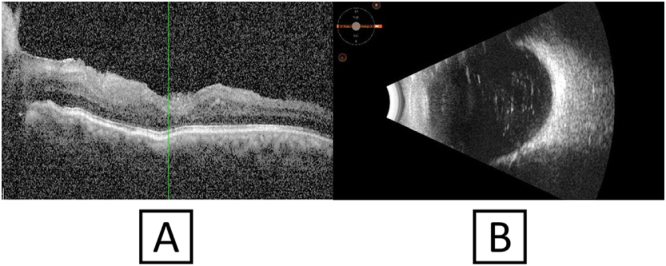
Multimodal imaging demonstrates improvement in hypotony-related posterior segment changes following the ligation of a Paul glaucoma implant tube. (A) Macular optical coherence tomography of the left eye shows improvement in macular folds secondary to hypotony following tube ligation. (B) B-scan ultrasonography of the left eye shows a flat retina and the absence of choroidal detachment.
